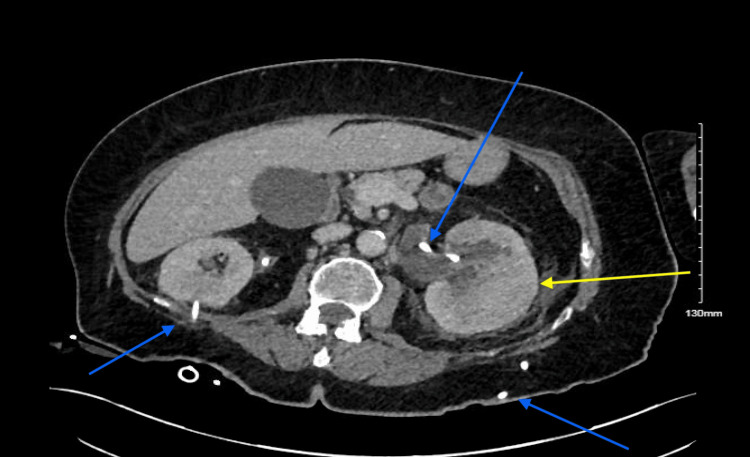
CT urogram performed three months before procedure demonstrating left-sided pyelonephritis (yellow arrow) and bilateral nephroureteral stents (blue arrows) in situ.
